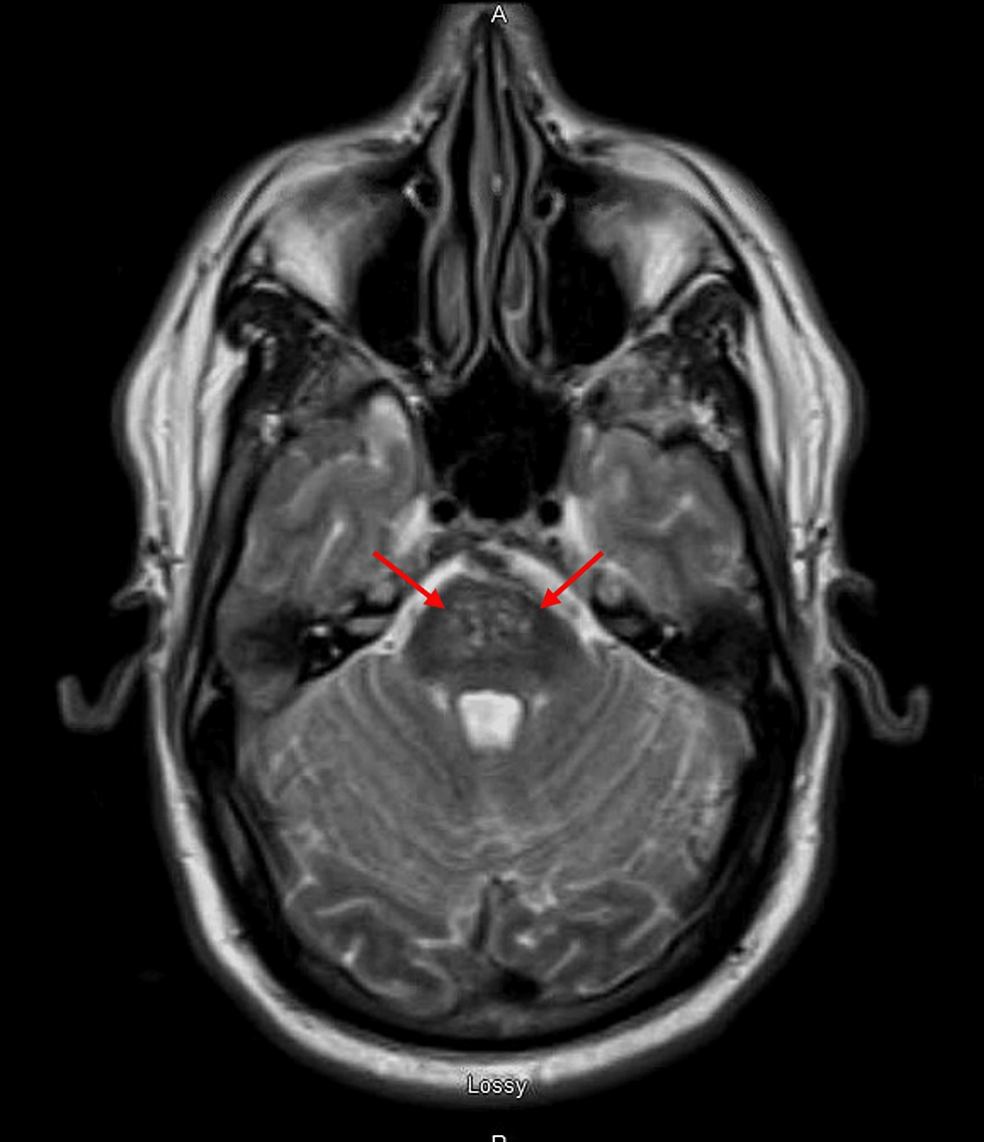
Arrows showing pontine hyperintensities in the initial T2-weighted imaging
